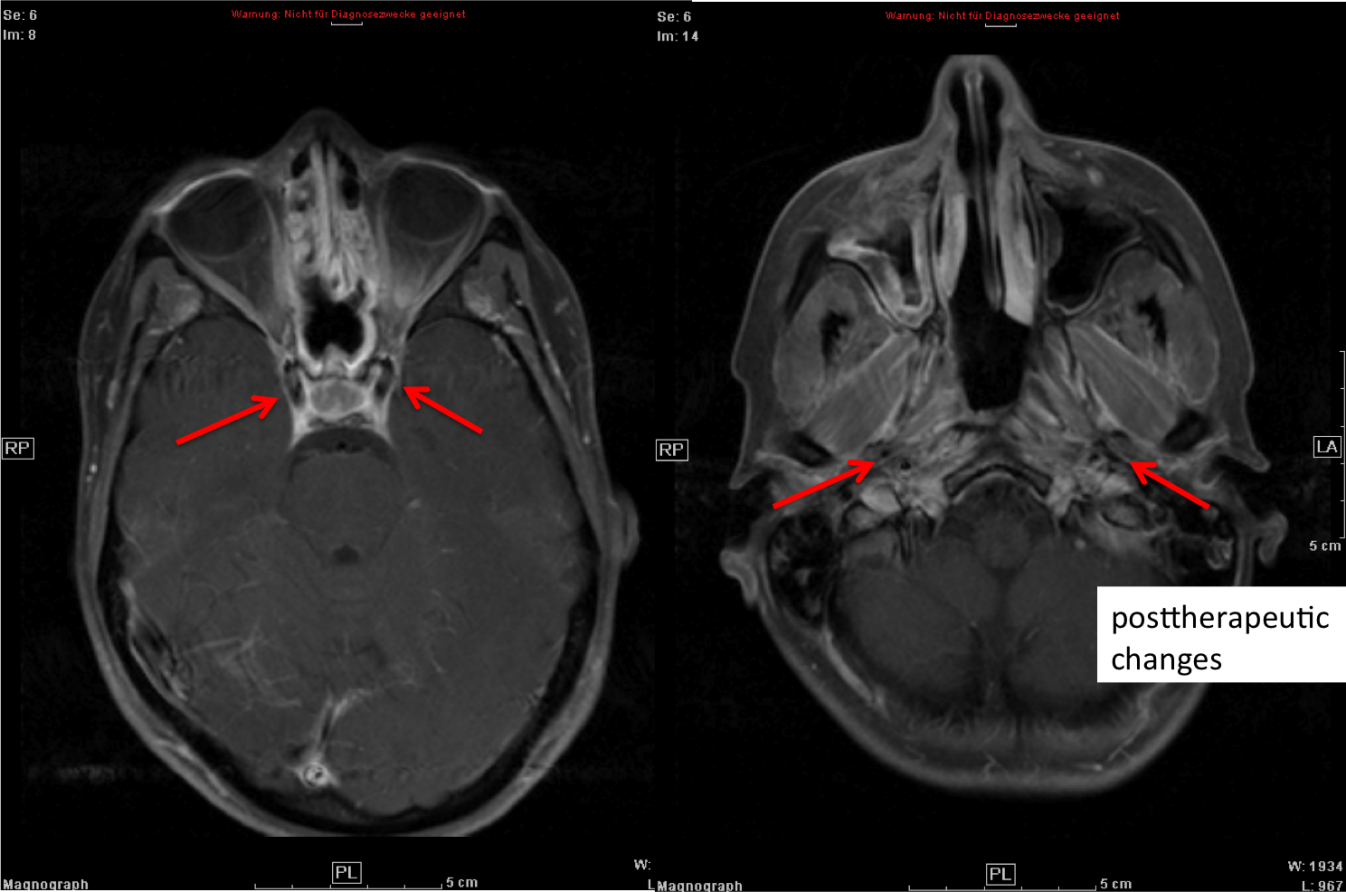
complete remission 6 months post combined RT (transversal contrast-enhanced MRI).
